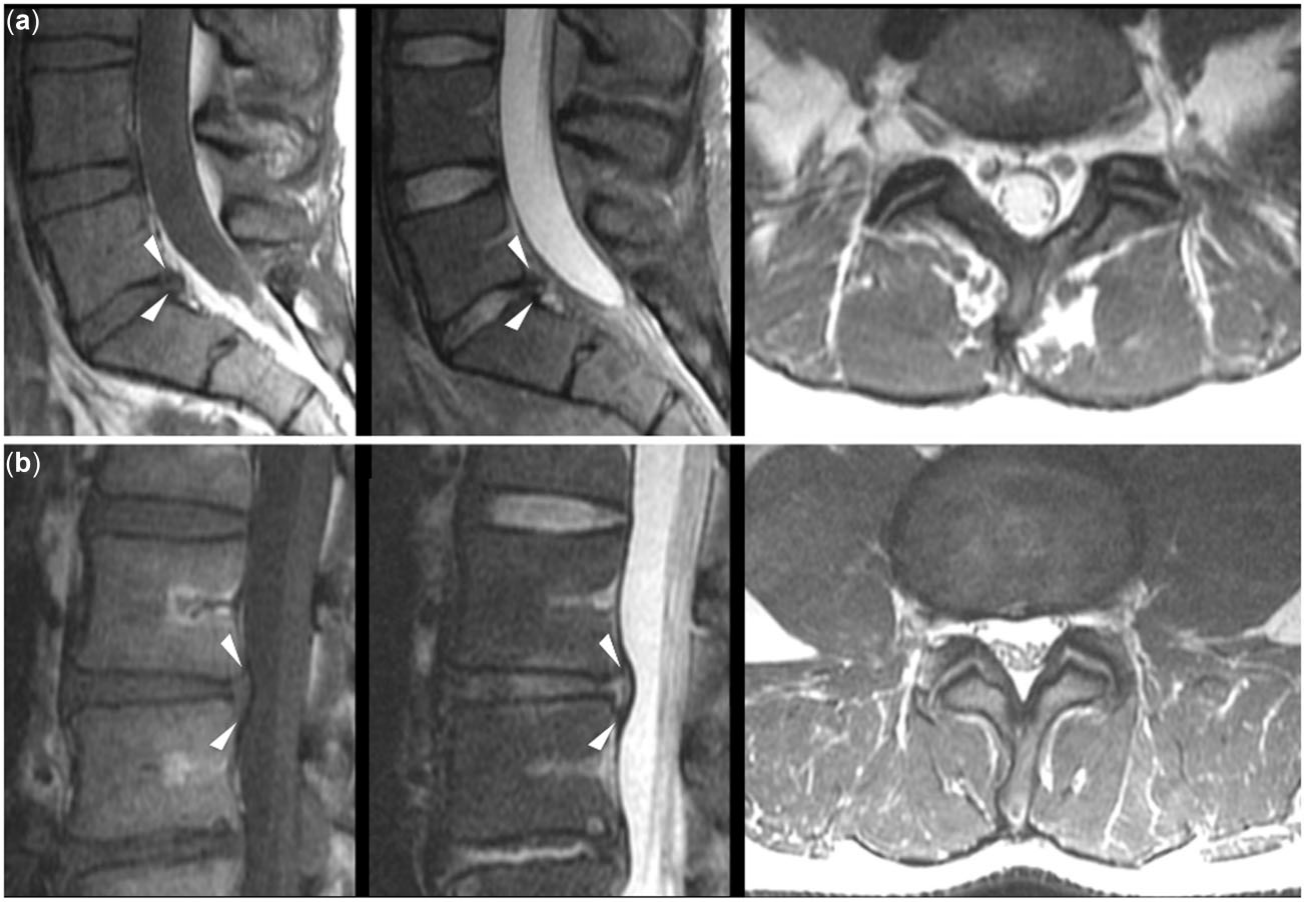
Disc herniation. Example cases for disc protrusion (a; arrowheads) and disc extrusion (b; arrowheads) on sagittal T1- and T2-weighted sequences and axial T2-weighted sequences. A disc protrusion is considered in case of a focal herniation of disc material beyond the margins of the adjacent vertebral bodies, while a disc extrusion is a focal herniation of disc nuclear material through an annular defect. In both presented cases, absent to minor resulting constriction of the thecal sac is shown on axial sequences.
